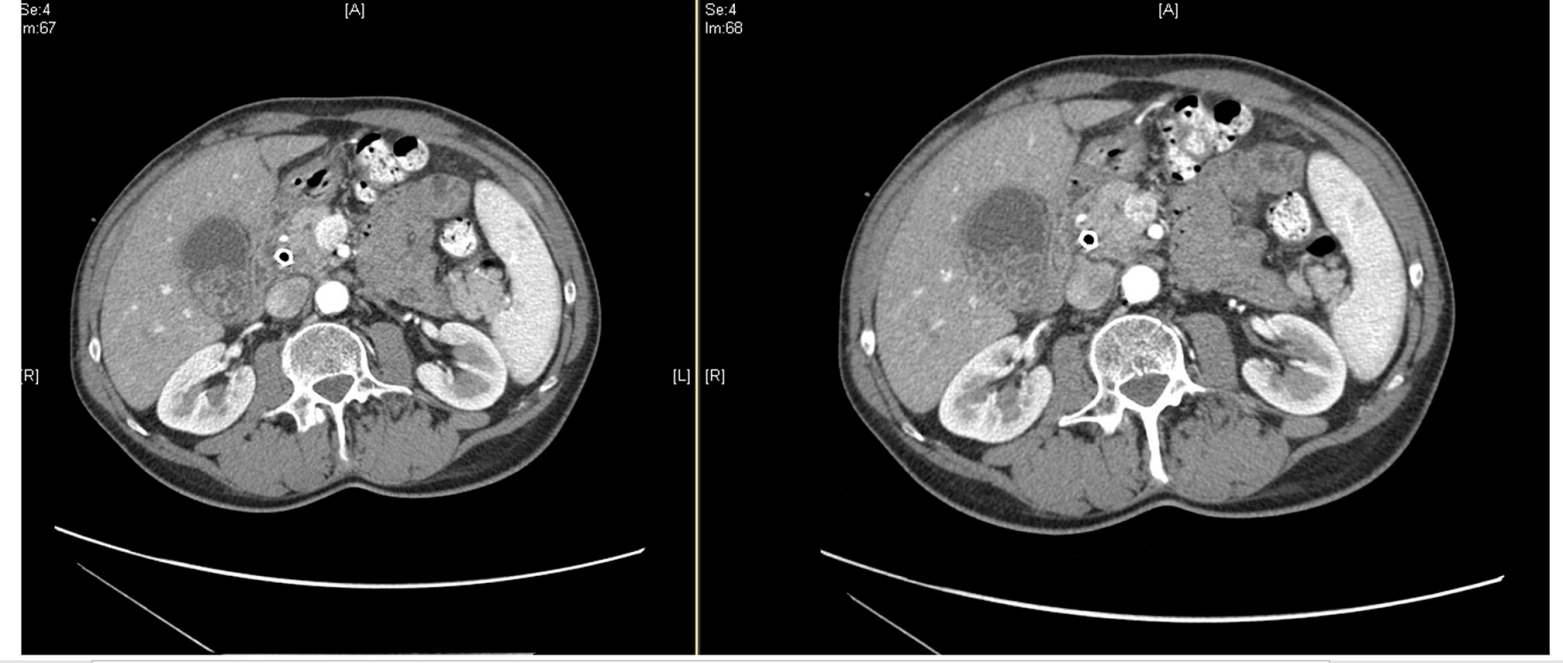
Abdominal CT scan: multiple cholelithiasis with acute cholecystitis.
